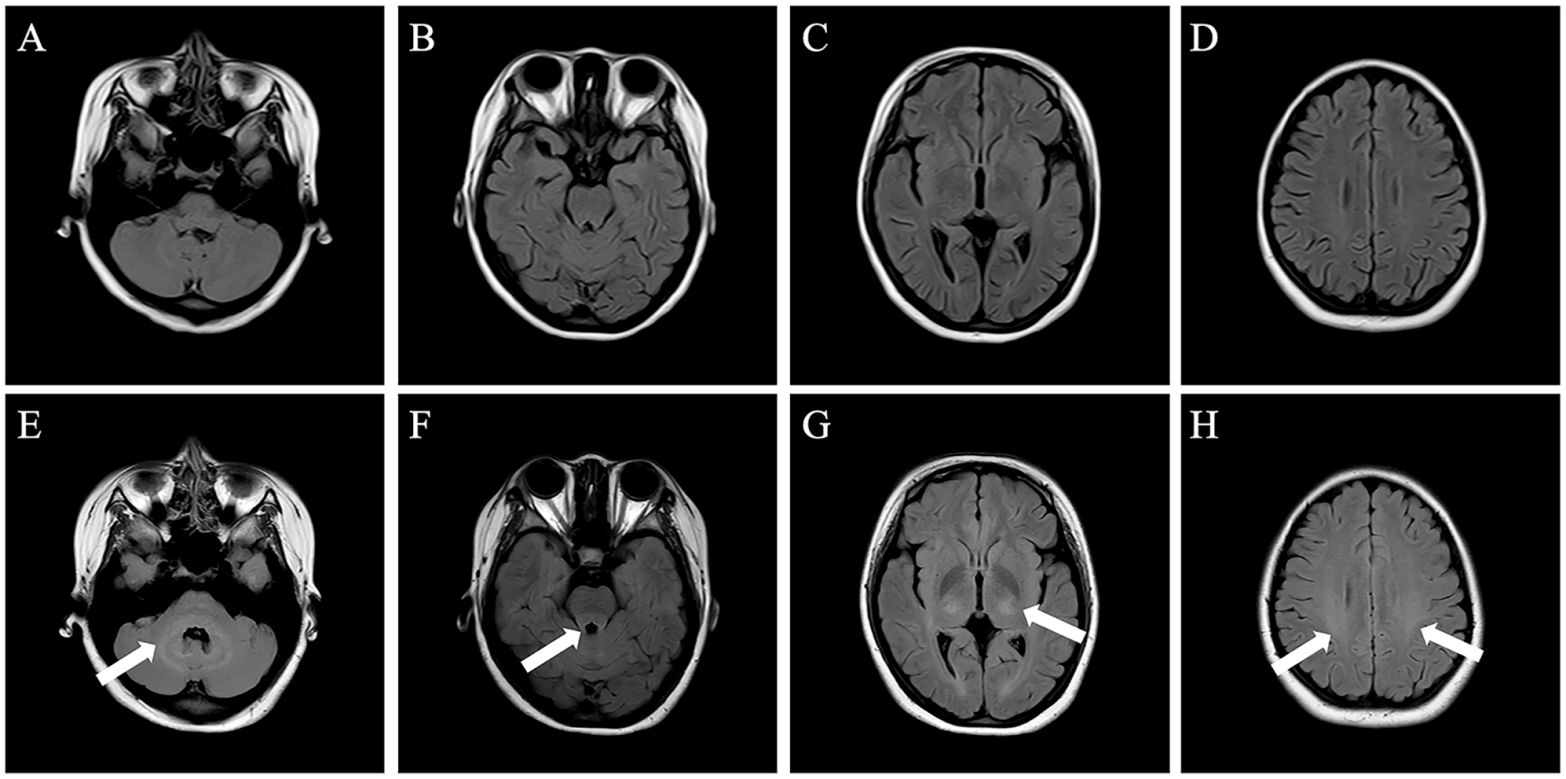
Initial and follow-up brain MRI (axial FLAIR sequence). (A–D) The initial brain MRI showed no structural abnormalities. (E–H) The follow-up brain MRI showed mild hyperintensities in bilateral cerebellar hemispheres (E), dorsal aspect of the brainstem (F), bilateral thalamic regions (G), and along the corticospinal tract pathways (H).
